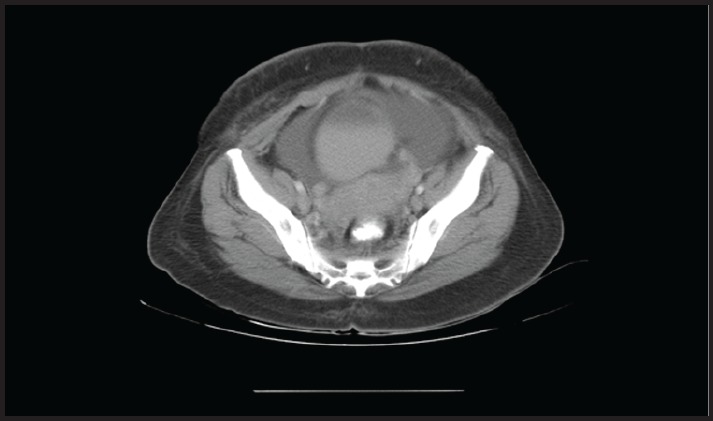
CT image showing adnexal mass.
